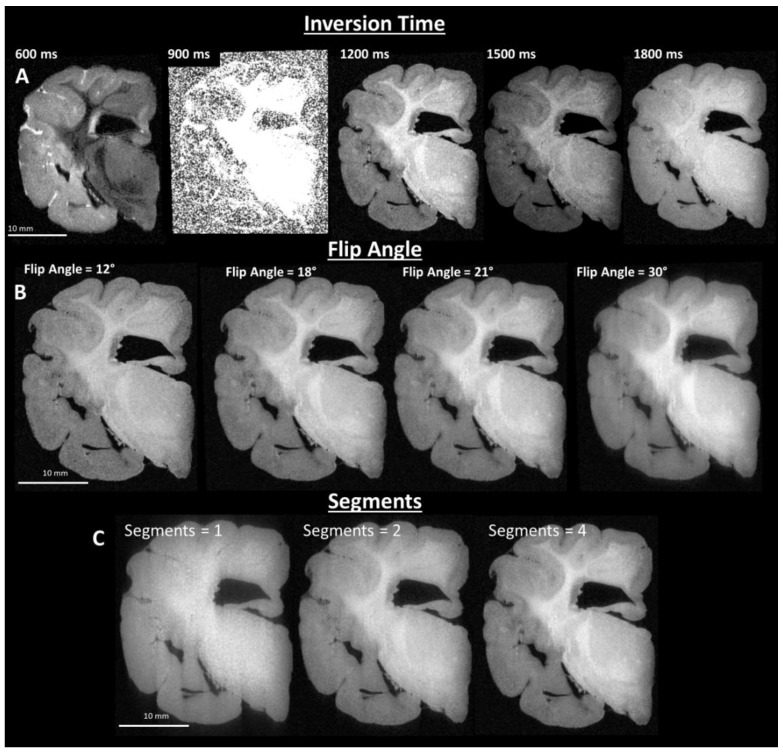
Imaging parameter optimization of T1-weighted images. (A) Inversion time optimization results in optimal image contrast between grey matter and white matter, by systematically varying the time delay between inversion of the MRI signal and signal acquisition (top left corners of image). (B) Imaging flip angle optimization results in adjustment of the image signal-to-noise and contrast by systematically varying flip angle. (C) Imaging sequence optimization of segmentation is varied to maintain the T1 weighted contrast produced by the initial signal inversion and delay to signal acquisition, collection of the data is segmented, e.g., for one segment a single inversion pulse is followed by signal acquisition in one 2D plane of k-space, whereas for four segments, following each inversion pulse, one-quarter of a 2D plane is acquired, sequentially.
